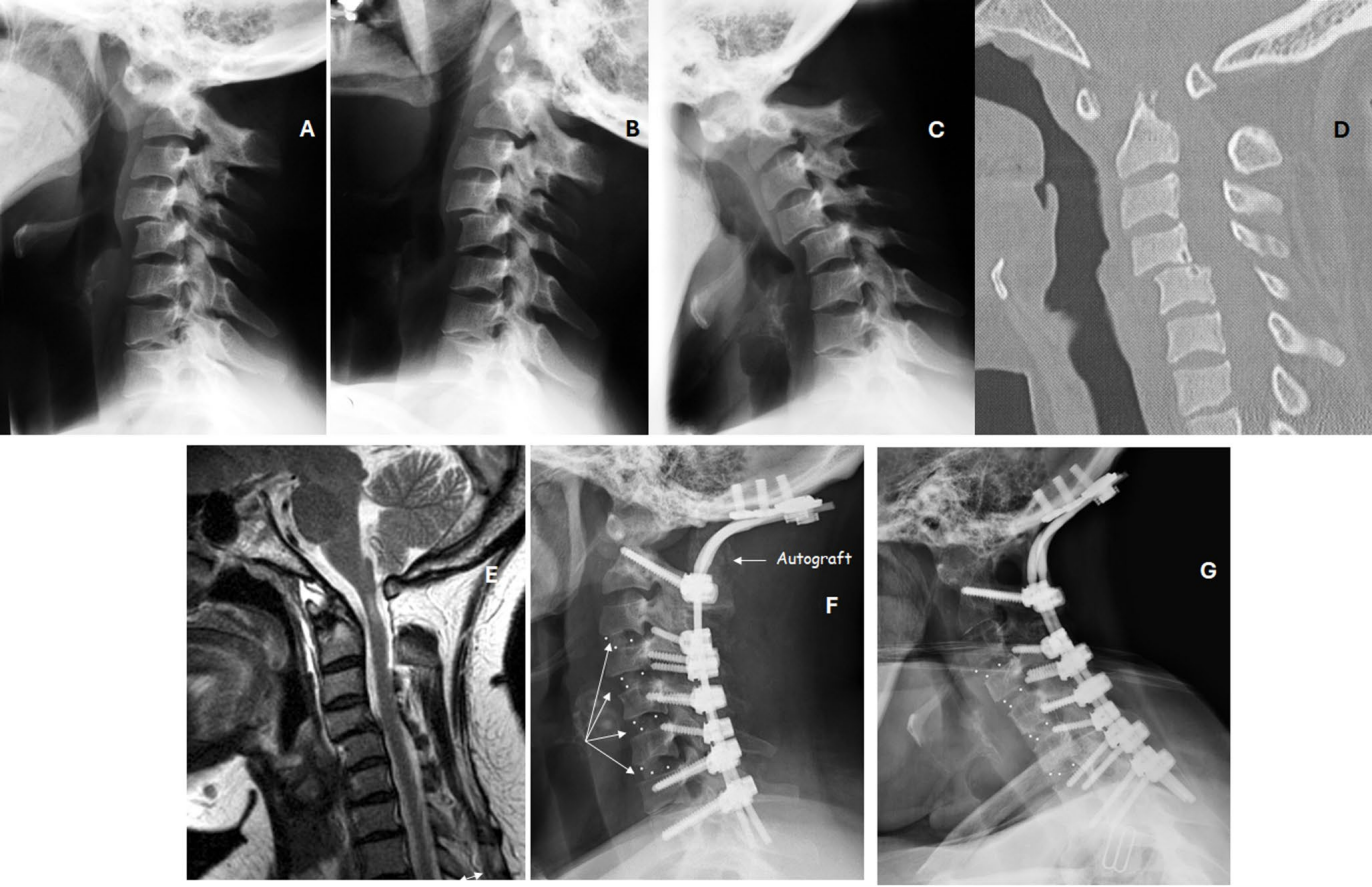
Case 7 Table 3. A-B and C: 35-year-old female with reducible Atlanto-Axial subluxation and non-reducible C4-C5 subluxation in flexion-extension radiographs, with neurological affectation Grade III in the Ranawat classification; D: CT scan; E: MRI in which severe myelopathy signal at C1-C2 level; F: Lateral radiography after a double approach, first posterior, occipito-T1 fixation with correction of the deformity (white arrows shows cages with autograft at C3-4-5-6-7 after discectomy); Autograft at Occipit-C2; G: Lateral XR eleven years after index operation showing slight kyphosis at cervicothoracic level
